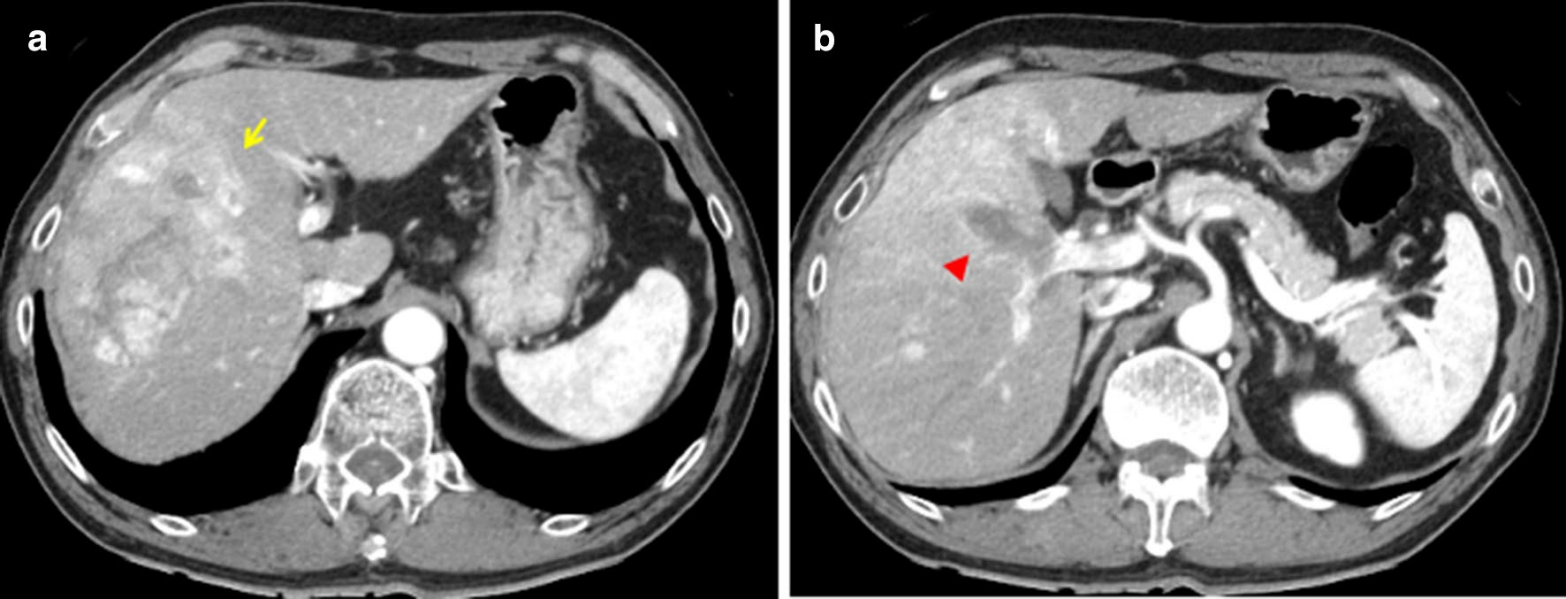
Abdominal enhanced CT findings at the presentation of the patient. a CT showing 10 × 7 × 7 cm-sized early enhancement and later washout tumor in the right lobe (yellow arrow). b CT showing portal vein invasion or tumor thrombus in the right portal vein (red arrowhead). *CT* computed tomography
